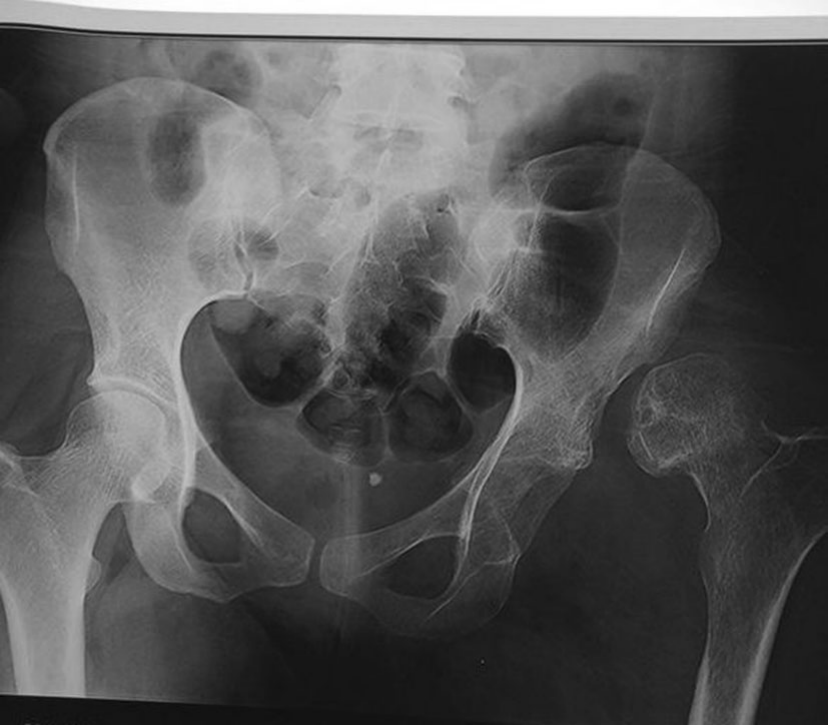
Preoperative X-ray of pelvis with neglected hip dislocation with false hip joint
